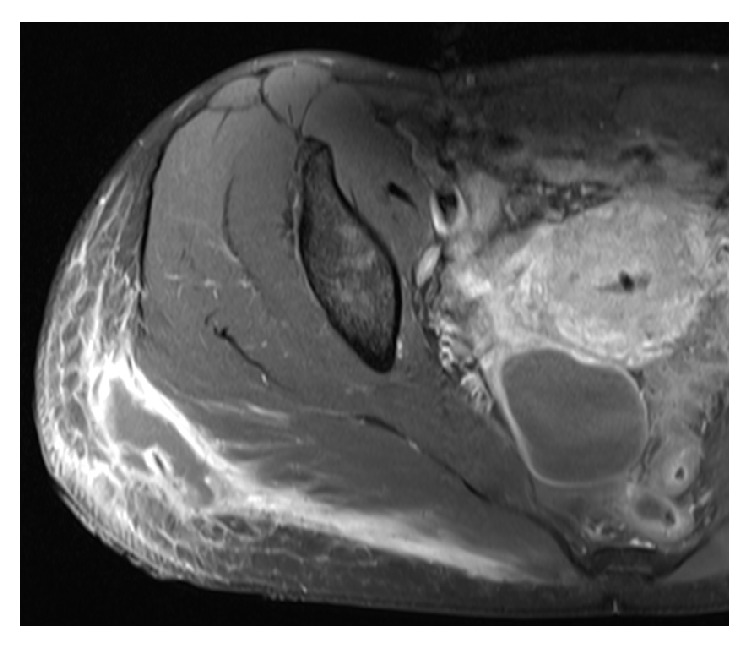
MRI shows T1 phase with gadolinium contrast, showing a small superficial gluteal collection joining large deep subcutaneous collections in contact with the muscular fascia, associated with diffuse infiltration of the neighbouring subcutaneous tissue and the gluteus maximus muscles.
